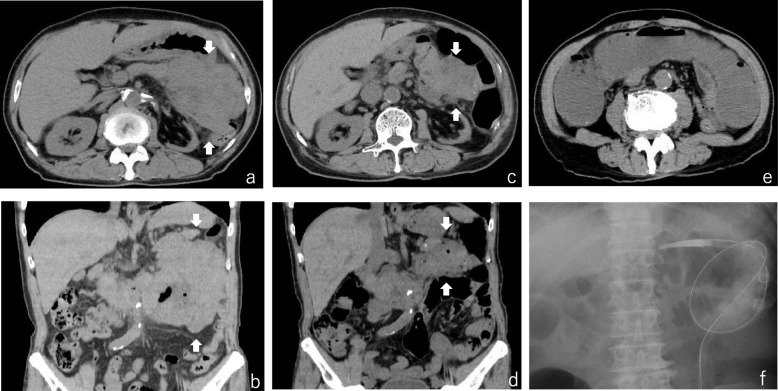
Abdominal computed tomography imaging. A plain abdominal computed tomography (CT) image on admission shows an irregular tumor in the left upper quadrant corresponding to the transverse colon. The tumor was in close contact with the pancreatic body and tail, and the gastric corpus greater curvature. Mildly enlarged lymph nodes were observed at the periphery of the tumorous lesion (1a and 1b). The second plain abdominal CT conducted 36 days after the first CT showed that the tumor had regressed spontaneously and the swelling of lymph nodes around the tumorous lesion had disappeared (1c and 1d). CT conducted 48 days after the first visit revealed intestinal distention on the oral side of the narrowed area in the transverse colon, and the patient underwent decompression by transanal ileus tube insertion (1e and 1f)
